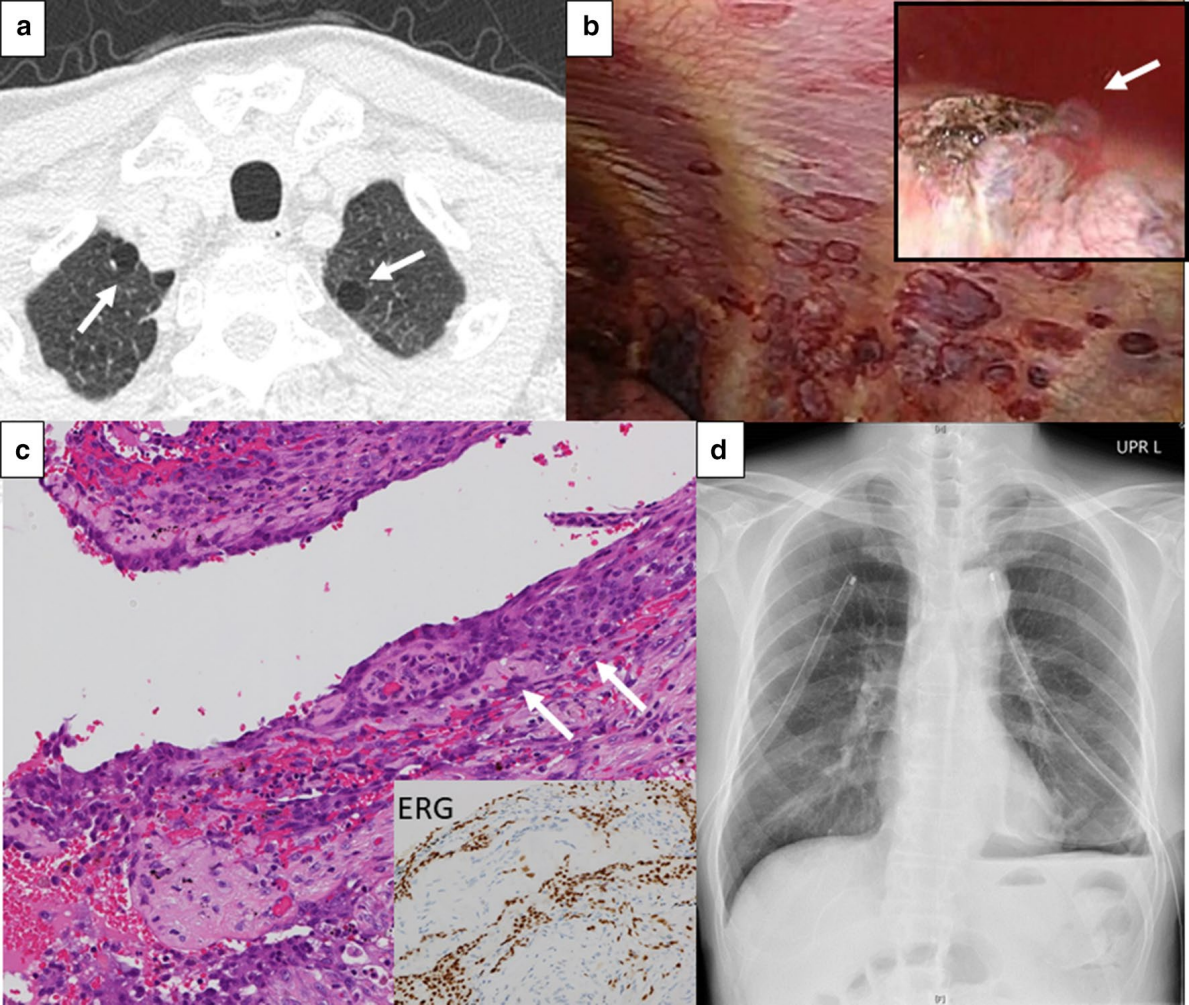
a CT, performed 1 week before diagnosed with pneumothorax, showed bullae lesions in the apex (arrows). b Intraoperative findings demonstrated bulla in the left apex (arrow) and numerous dark-red spots in the parietal pleura. c Pathological findings showed atypical spindle-like cells positive for ERG infiltrating the visceral pleura (arrows). d Chest X-ray showed expansion of the bilateral lung; however, pleurodesis was ineffective
